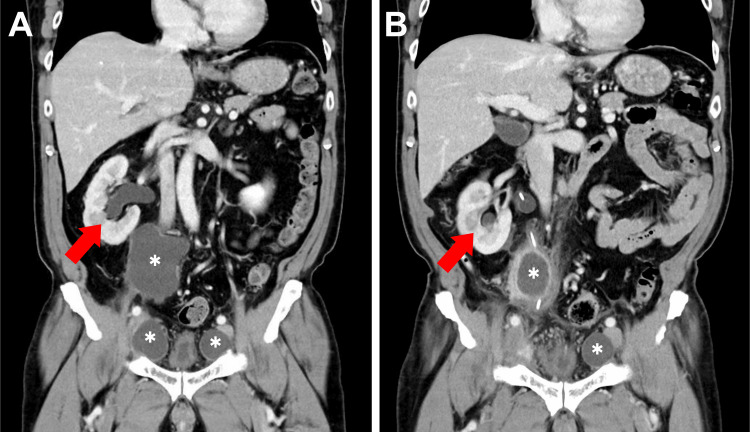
Contrast-enhanced CT images in coronal view demonstrating the regression of lymphoceles and improvement in hydronephrosis over time (A) One month after RaRP with pelvic lymph node dissection, there is significant right hydronephrosis (red arrow) and multiple lymphoceles (white asterisks). (B) Three-month follow-up scan showing a significant reduction in the size of the lymphoceles (white asterisks) and partial resolution of right hydronephrosis (red arrow). CT: computed tomography; RaRP: robotic-assisted radical prostatectomy
